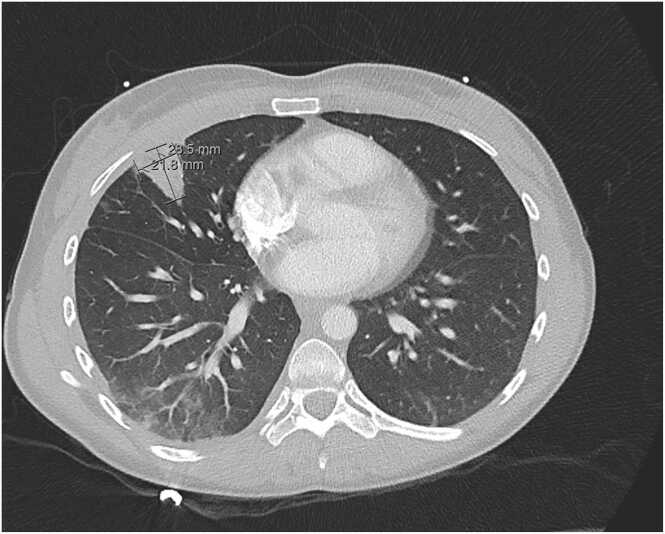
Computed tomography image with a wedge-shaped right middle lobe lung nodule.
